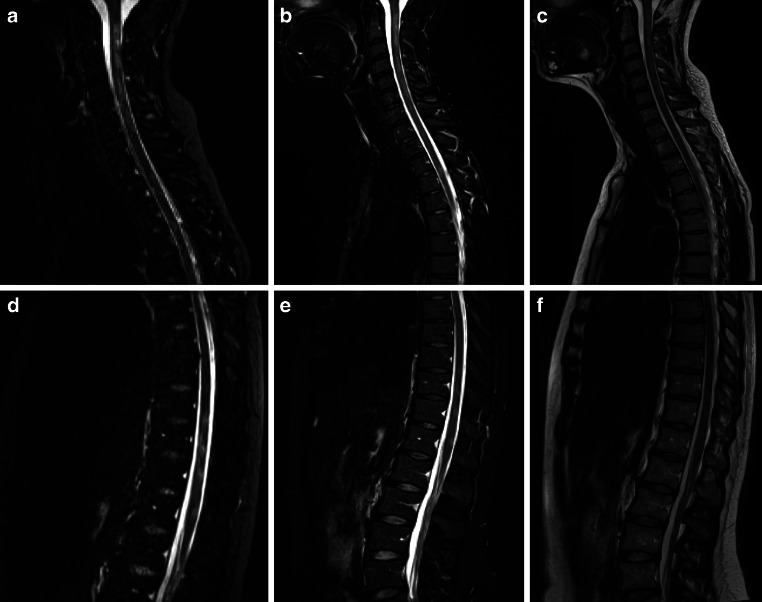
Exemplary acquired datasets with three different MRI techniques (PSIR, STIR and T_2_-w) in a 37-year-old female with a 15-year history of multiple sclerosis (MS) and an expanded disability status scale (EDSS) of 2. The spinal cord was examined with two stacks to cover the cervical and upper thoracic spinal cord (a–c) and the middle and lower thoracic spinal cord (d–f). MS lesions of the cervical and thoracic spinal cord are visible in the magnitude-3D-PSIR-MRI-images (a and d), the STIR images (b and e) and the T_2_ weighted images (c and f)
